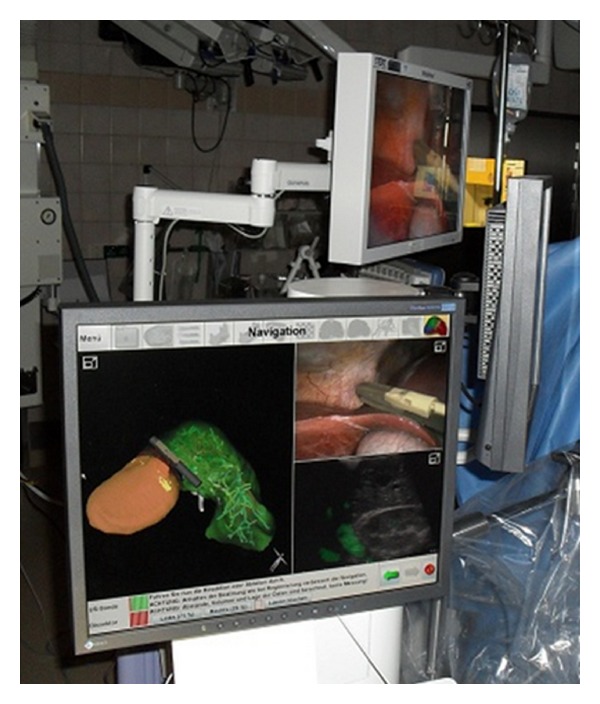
LapAssistent placed intraoperatively on the right patient side; simultaneous laparoscopic and ultrasound scenes are described above.
